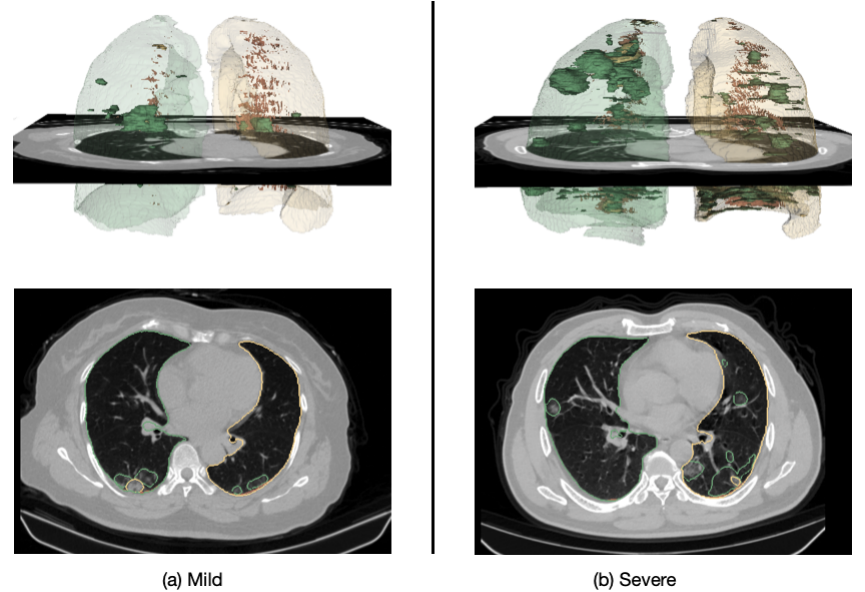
Representative chest computed tomography scans and the lung and lesion models of (A) a mild COVID-19 case and (B) a severe COVID-19 case.
